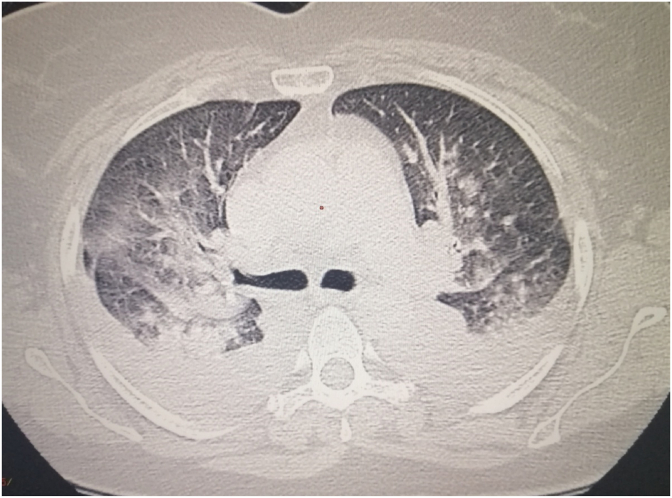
Thoracic CT scan showing 50% of lung damage.
